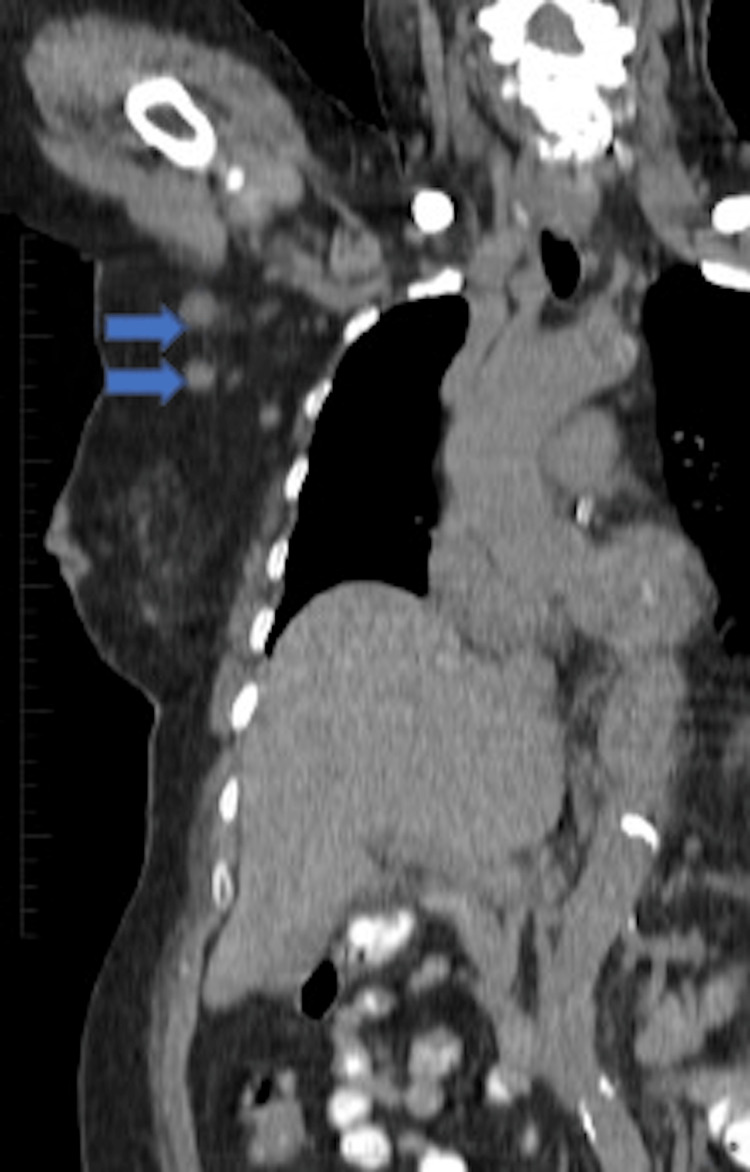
PET-CT in coronal view of the mid-thoracic/axillary region showing approximately 2 cm axillary lymph nodes. PET-CT: positron emission tomography-computed tomography
